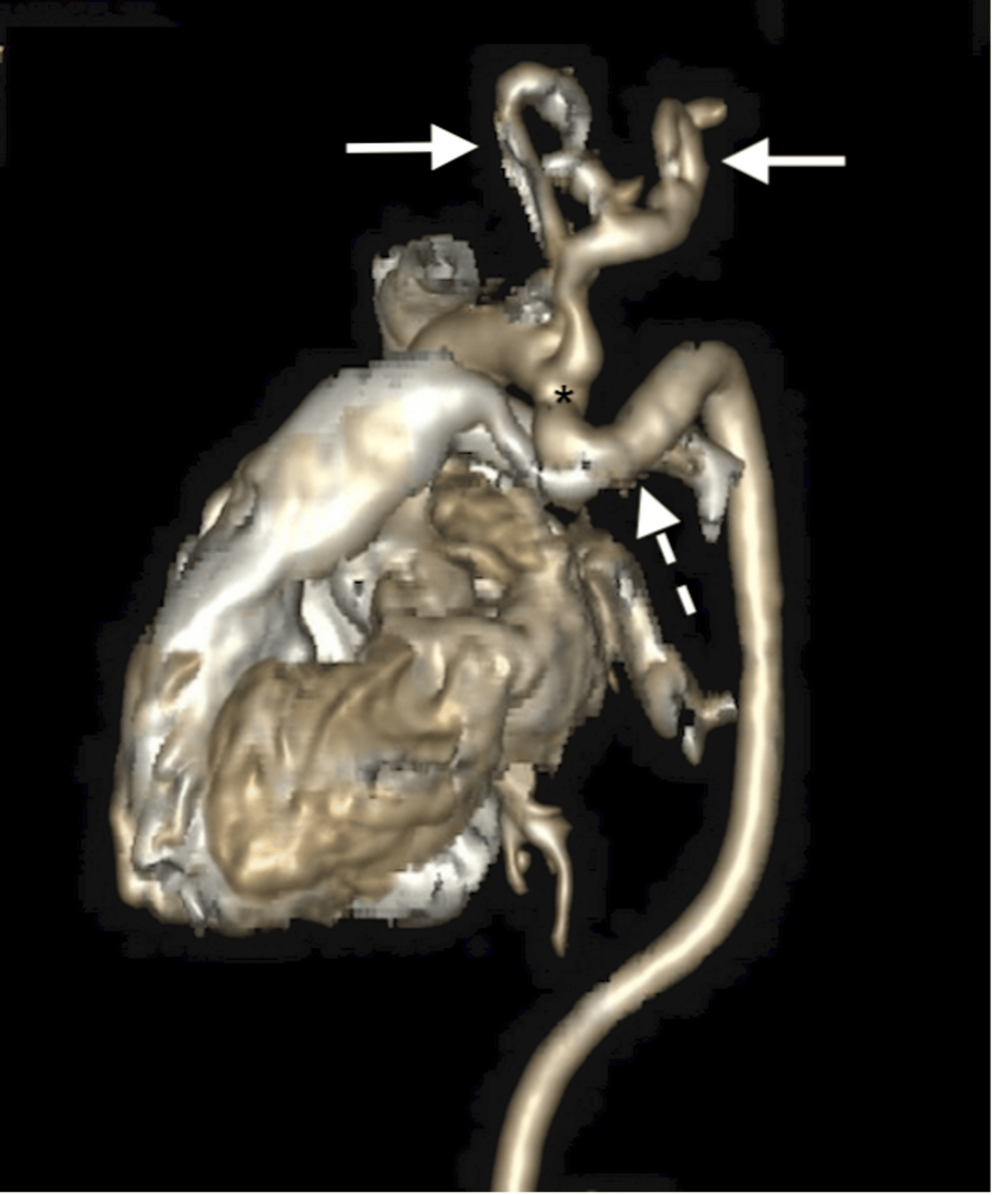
Volume-rendered CT. The image reveals a tortuous aortic arch (dashed white arrow) and its main branches (white arrows). Note the relatively narrow aortic arch just after the origin of the left subclavian artery (asterisk).
